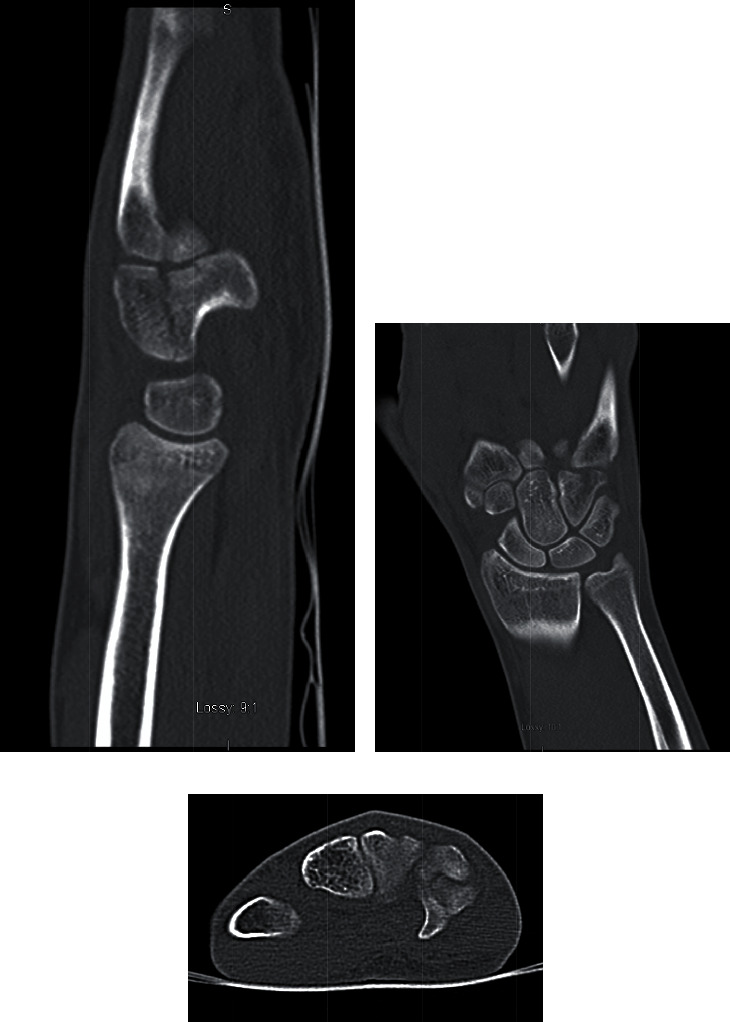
(a–c) CT images showing a longitudinal split fracture of both the hook and body of the hamate with 2 mm of dorsal displacement and intra-articular extension involving the CMC joint.
